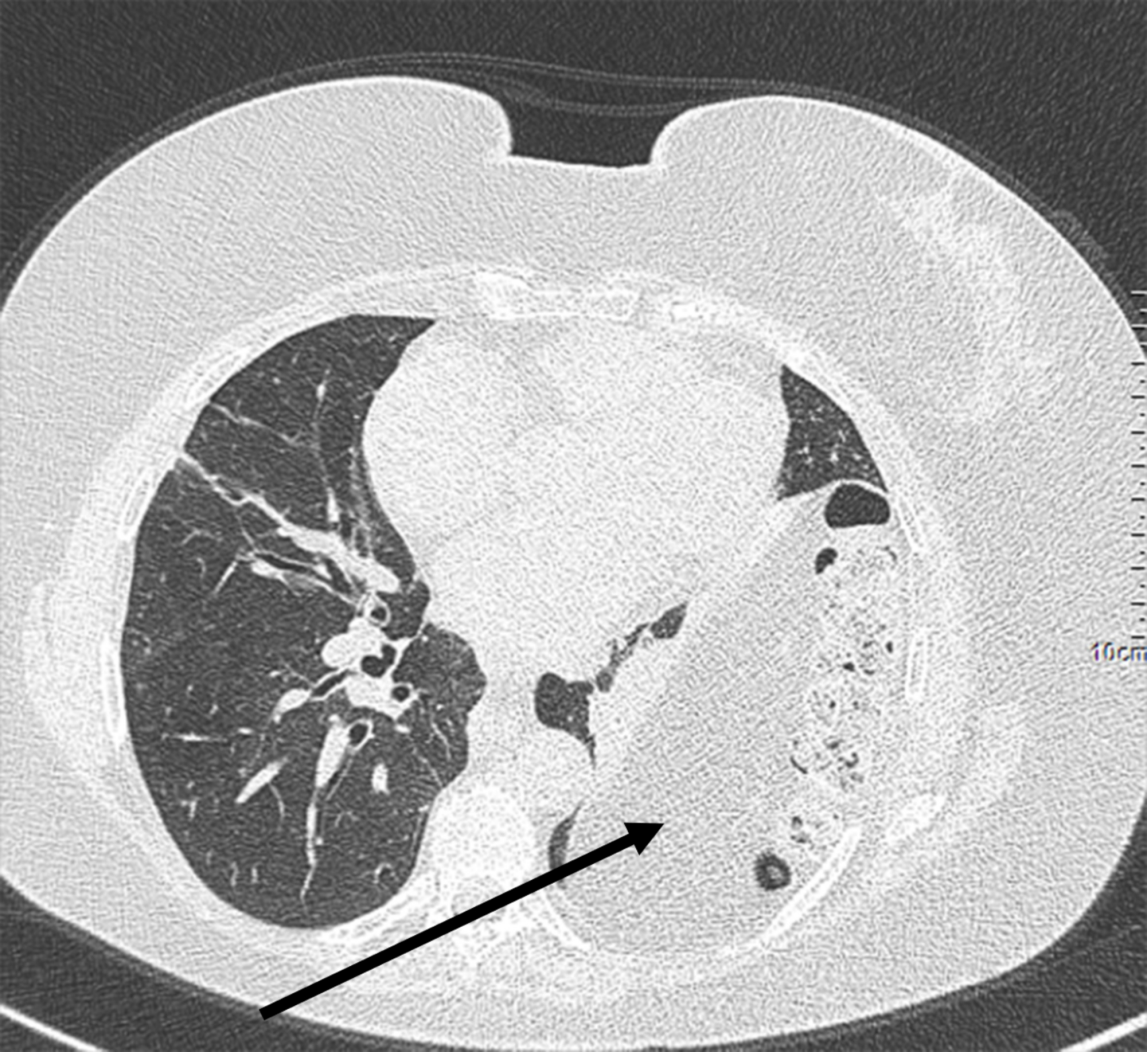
Thorax CT detected the splenic flexure and small intestine in the left chest, leading to mediastinal shift to the right CT, computed tomography
